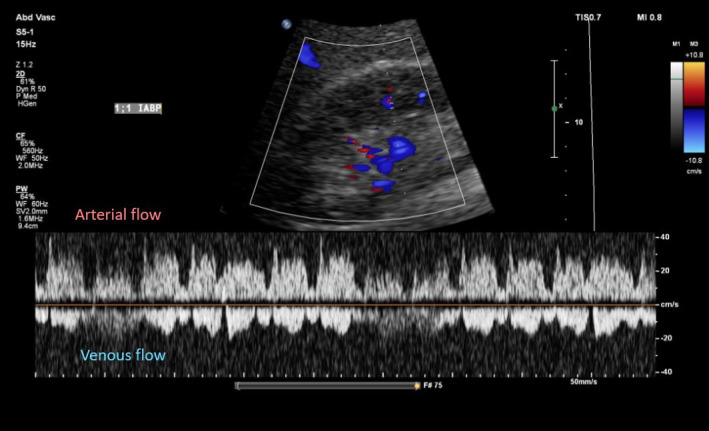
Intrarenal Doppler showing minor flow interruptions in the vein.
